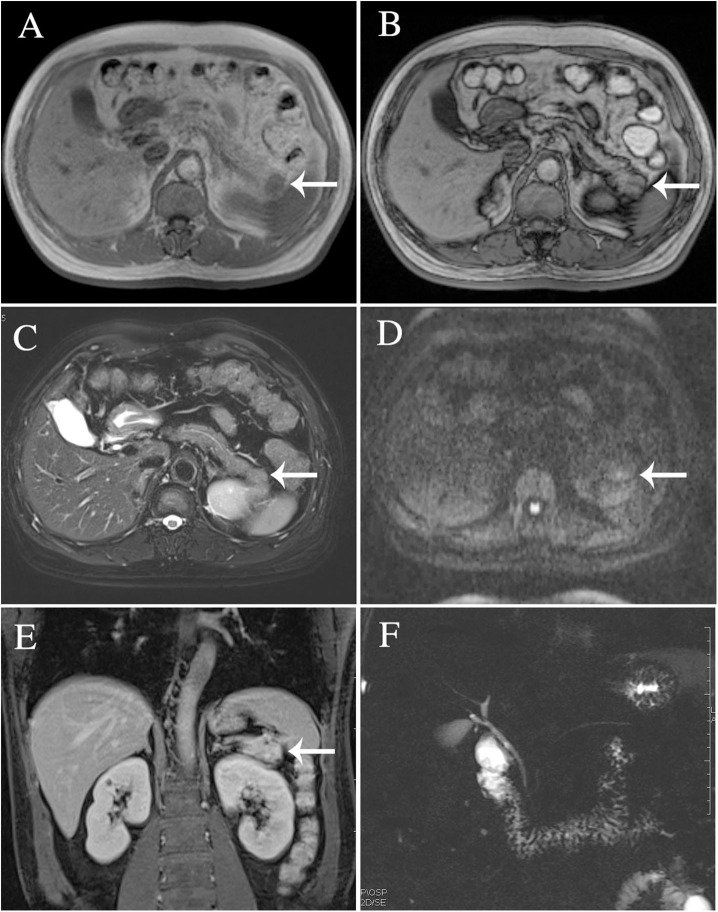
The lesion was identified in T2-weighted images (C), diffusion-weighted images (D), and magnetic resonance cholangiopancreatography (F). There was no significant difference between the in-phase (A) and the out-of-phase MRI imaging (B). Coronal MRI imaging (E).
